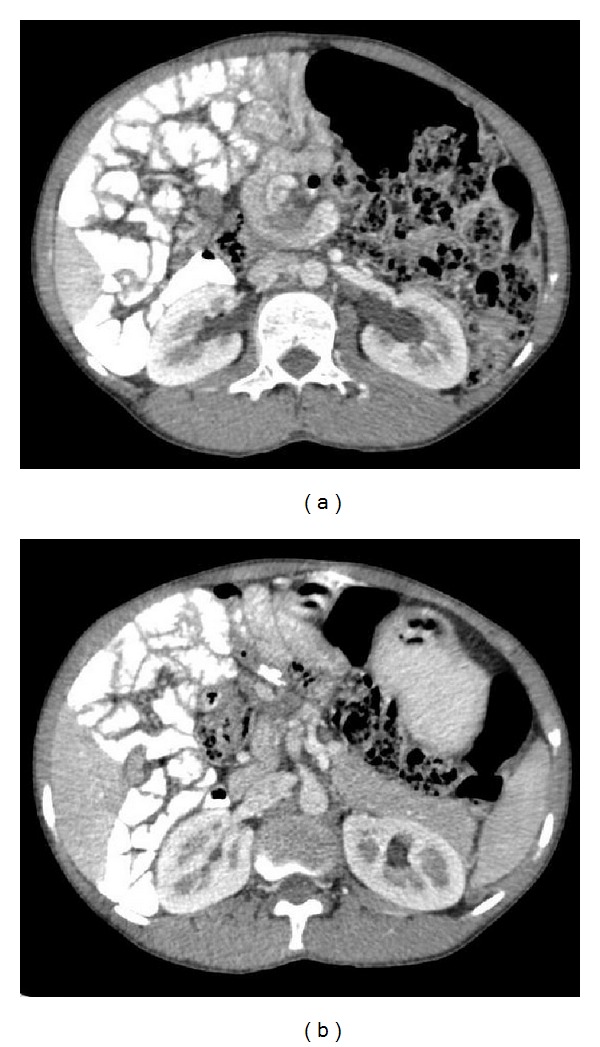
(a) Axial postcontrast CT image showing duodenum and SMV wrapping around axis of SMAin clockwise direction producing the characteristic whirl sign. Dilatation of SMV is also noted. Jejunal loops are seen of on right side of abdominal cavity. (b) Axial postcontrast CT image showing reversal of the normal anatomic relation of the SMAand SMV, that is, SMV is seen on the left side while SMA on the right side.
